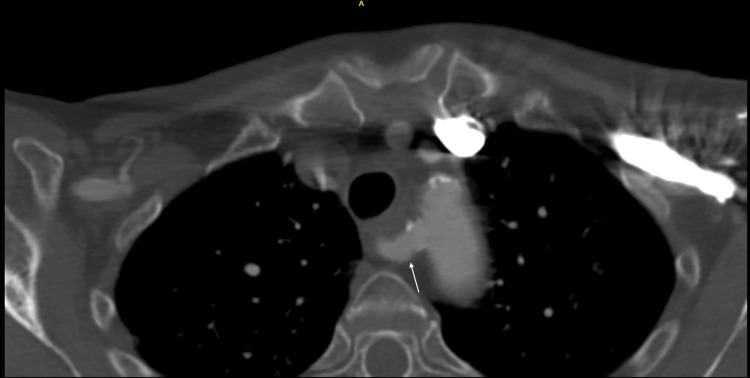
Axial view of CTA showing the posterior course of the retroesophageal ARSA (white arrow) CTA: Computed tomography angiography, ARSA: Aberrant right subclavian artery
